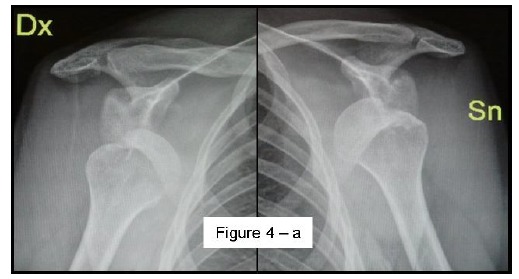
A case of bilateral engaging Hill-Sachs lesion on AP X-ray 108x58mm (150 x 150 DPI).
